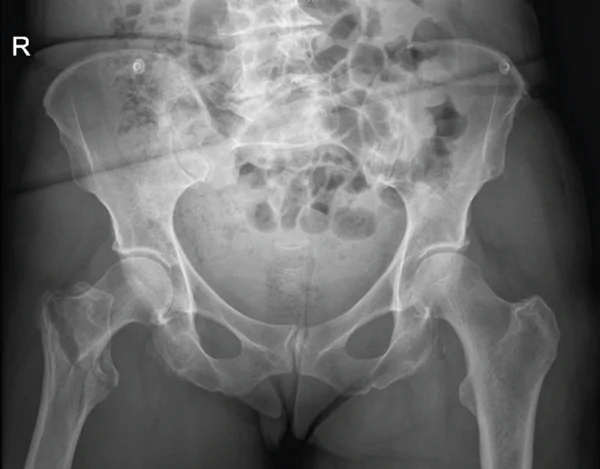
AP pelvic radiograph after initial injury showing right‐sided pertrochanteric hip fracture.
